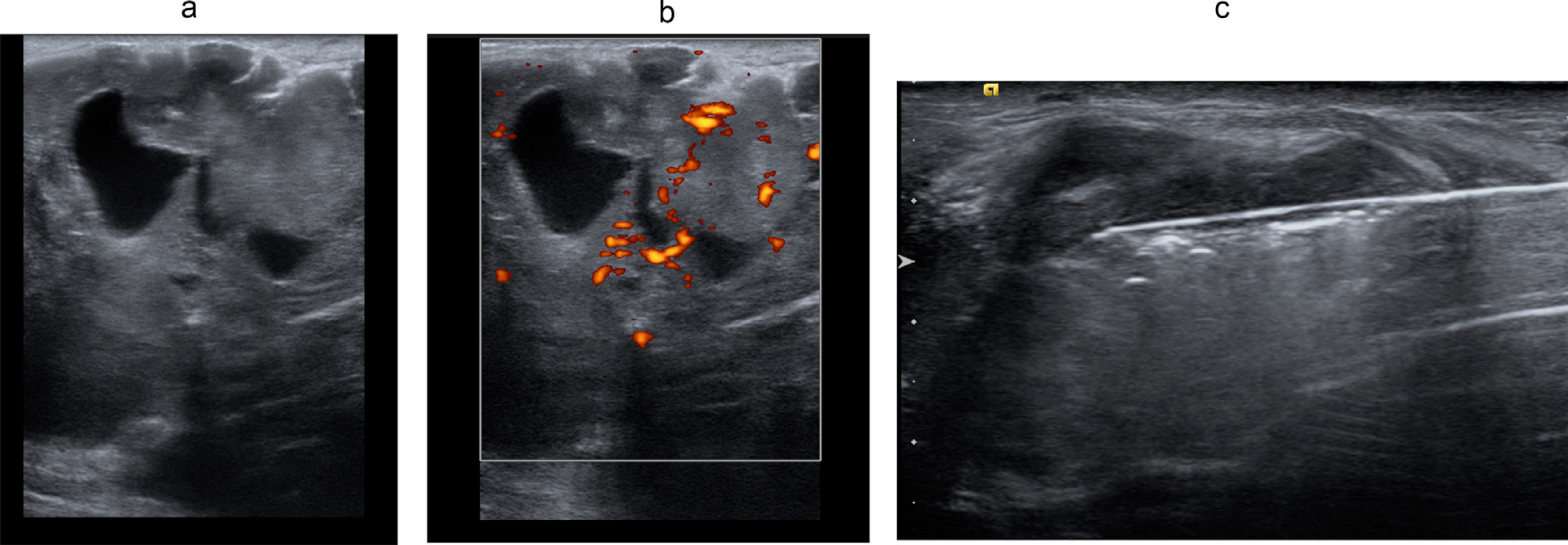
Ultrasound images of the right breast. A huge heterogeneous mass in the right breast (Left), with increased vascularity (Middle) and internal cystic areas. The border is circumscribed. Ultrasound-guided core biopsy performed using a 14G Maxcore needle (Right).
